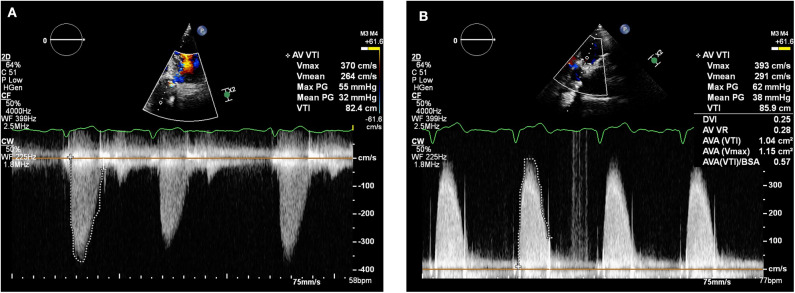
Transthoracic echocardiogram (August 2018): Continuous wave doppler in the apical 5 chamber view (A) and right parasternal long axis view (B) demonstrating a maximum transaortic velocity of 3.9 m/s.
